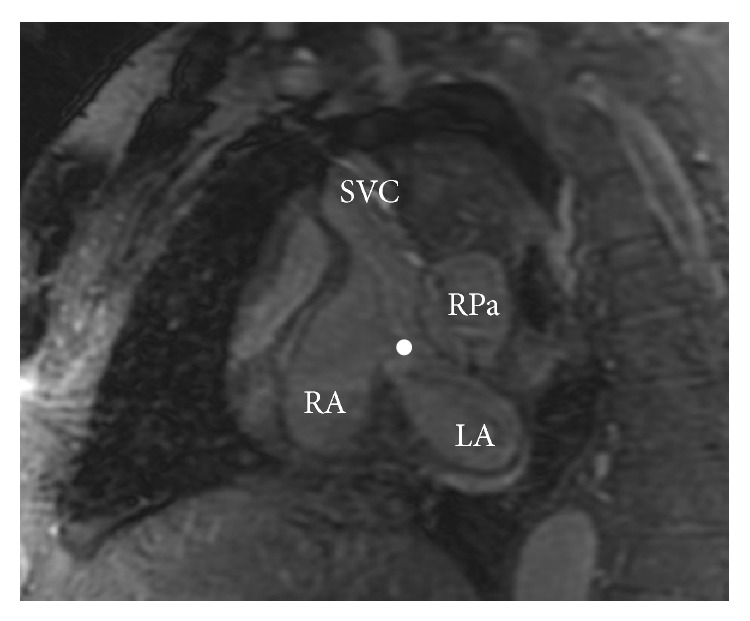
Sagittal 3-dimensional steady state free precession (SSFP) magnetic resonance image demonstrating a large sinus venosus defect (circle). SVC: superior vena cava; RPa: right pulmonary artery; RA: right atrium; LA: left atrium.
